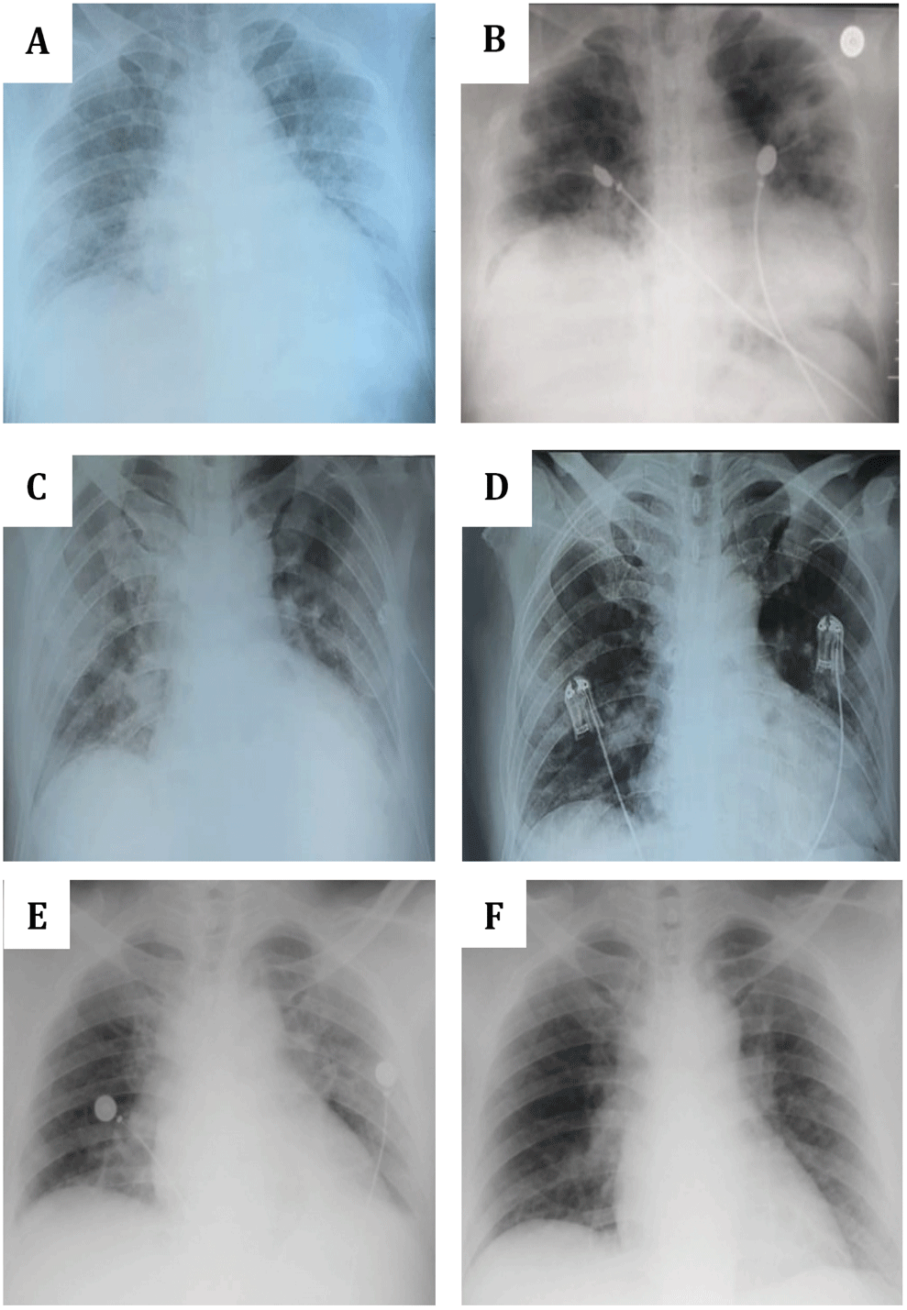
Chest X-ray of the three patients. (
